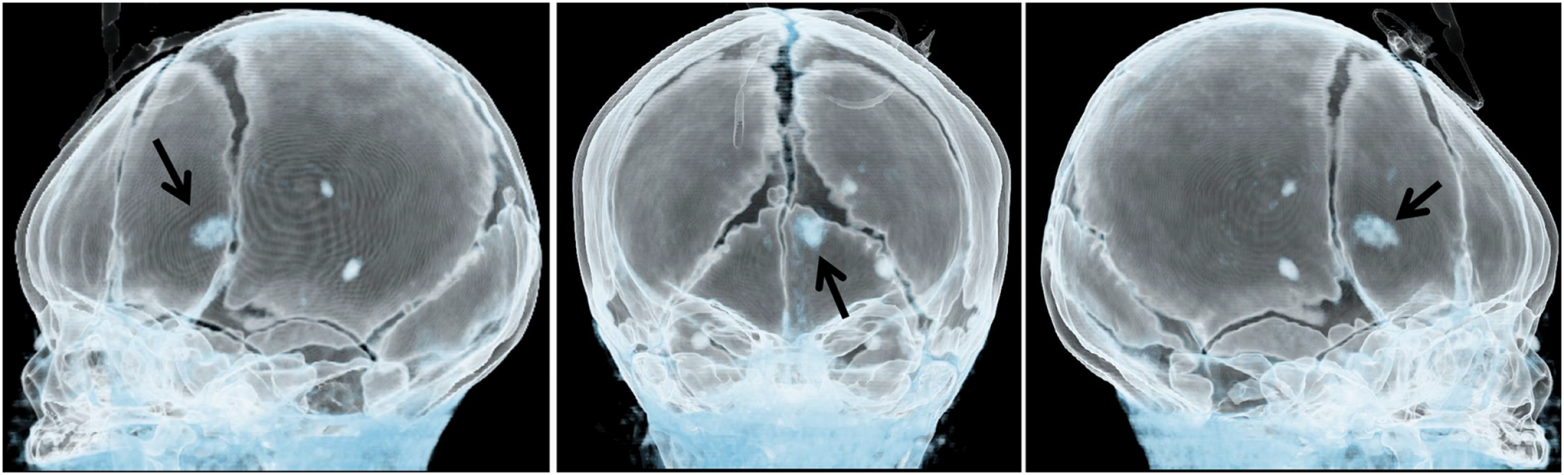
Three-dimensional cerebral computed tomography angiography showing multiple nodular high-density shadows with distinct borders. These shadows were distributed across several regions, including the left fronto-parietal lobe, right frontotemporal lobe, and bilateral subependyma of the lateral ventricles. A prominent lesion, measuring approximately 1.2 × 1.4 cm, was observed adjacent to the frontal horn of the right lateral ventricle (arrow).
